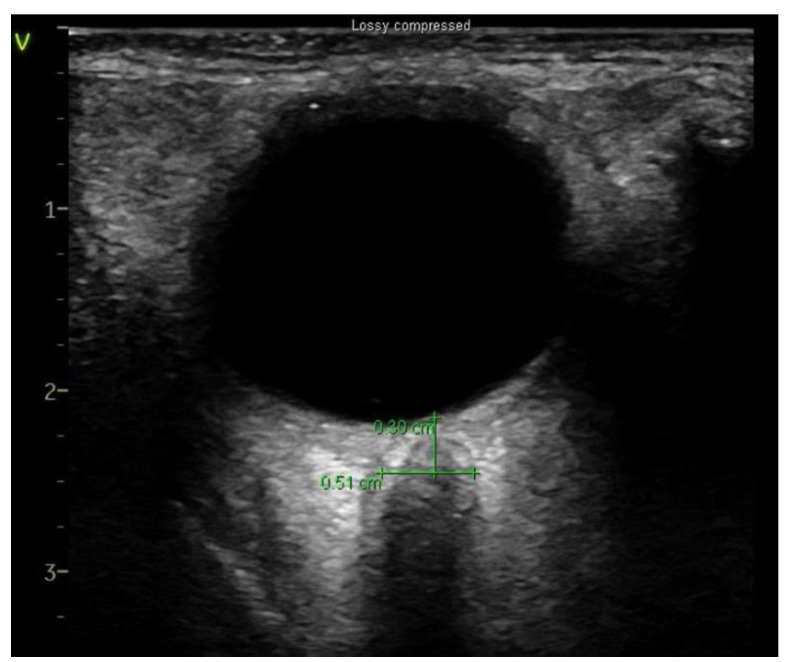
Sonographic optic nerve sheath diameter (ONSD).
